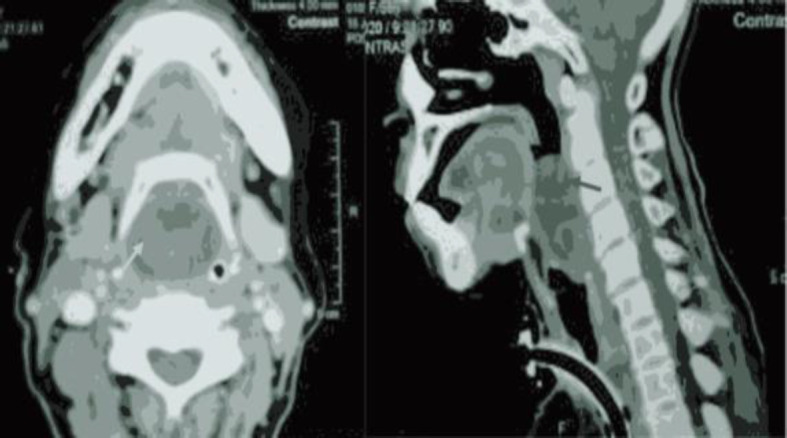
A computed tomography (CT) scan of the neck shows a round mass obstructing the larynx from the axial view (yellow arrow) and sagittal view (red arrow).
